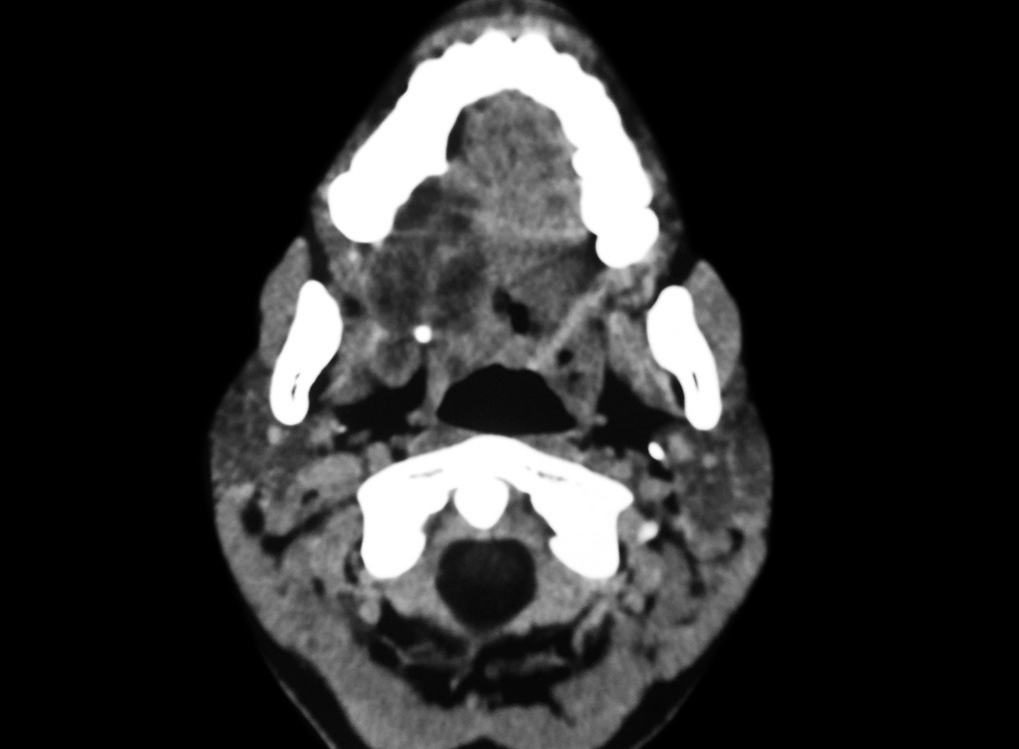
Computed tomography image of the lesion
